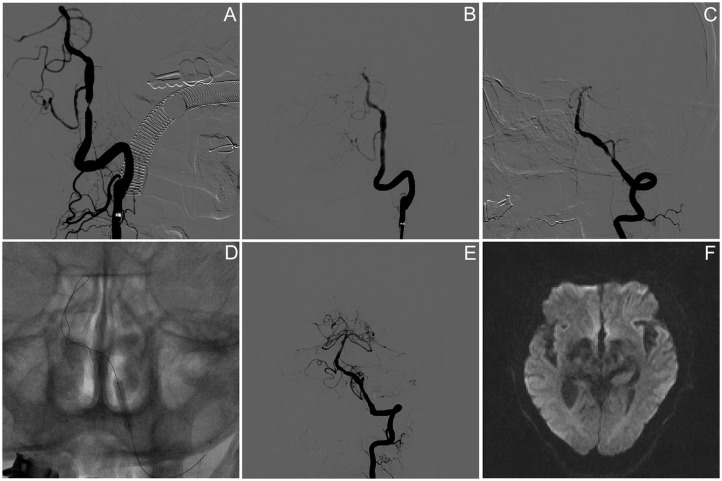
Paclitaxel-coated balloon angioplasty for intracranial sISR. (A) Symptomatic severe vertebral artery atherosclerotic stenosis despite of aggressive medical management. (B) Angiography results after PTAS using wingspan stent. (C) Symptomatic ISR because of the discontinuance of aggressive medical management. (D) Drug-coated balloon dilatation. (E) Angiographic result after the DCB angioplasty. (F) Brain DWI result on day 5 after procedure presented no new infarction.
